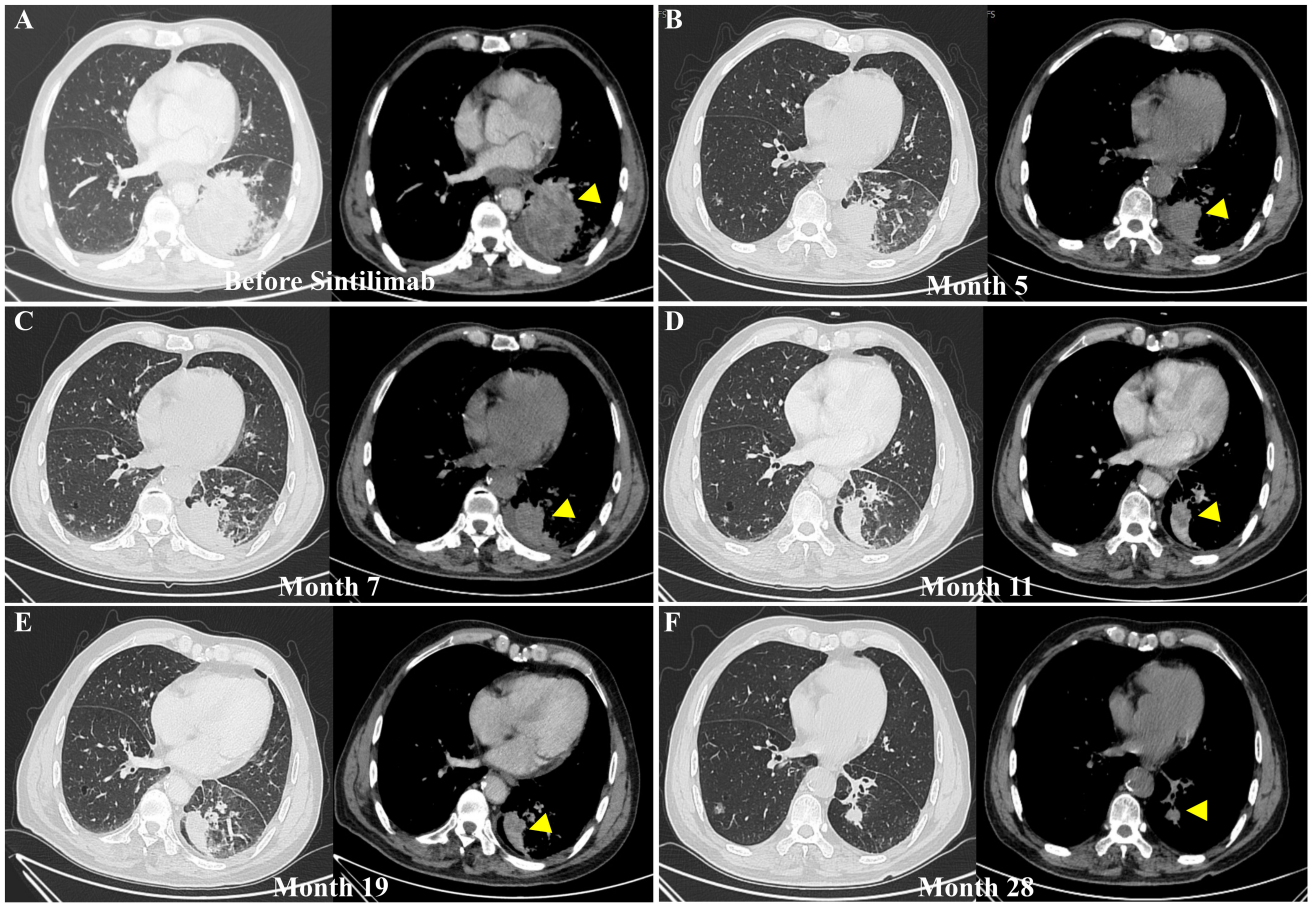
Longitudinal assessment of therapeutic response via contrast-enhanced computed tomography (CT) scans. (A) Baseline scan (Month -1.2) prior to sintilimab initiation, demonstrating a large, irregular, heterogeneously enhancing solid mass in the posterior basal segment of the left lower lobe, measuring approximately 13mm*13mm (yellow triangles). The lesion exhibits proximity to the visceral pleura on the mediastinal window. (B) Initial therapeutic response at 5 months of sintilimab and apatinib therapy, showing a marked reduction in tumor volume. (C–E) Sustained remission during maintenance therapy. (F) Pre-admission scan at 28 months of treatment, displaying the tumor status prior to the onset of the metabolic crisis. The left panel of each pair represents the lung window, and the right panel displays the corresponding mediastinal window.
